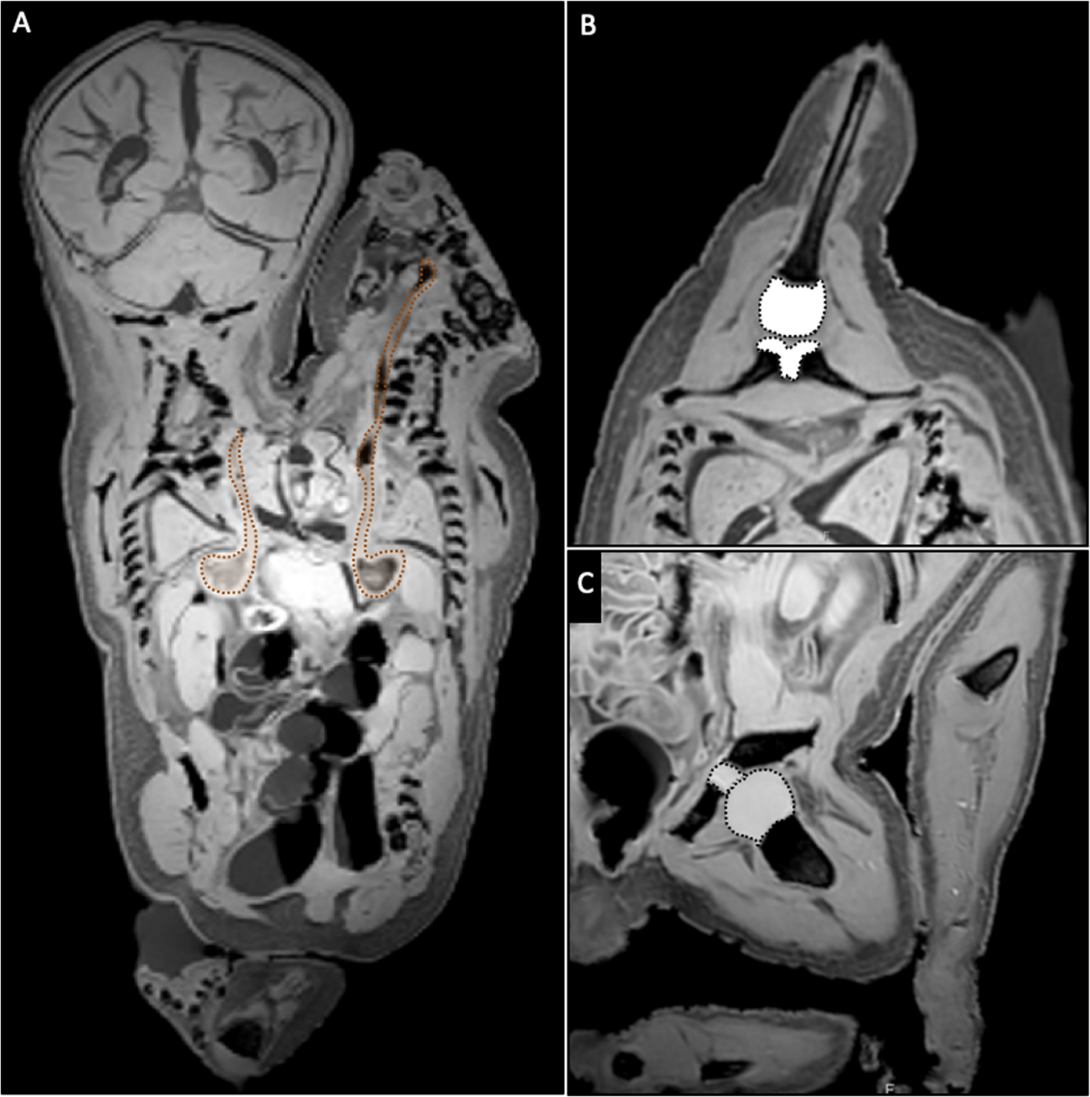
a Coronal T1-weighted magnetic resonance (MR) image showing two separate esophagi and mirror-imaged stomachs underneath an overarching diaphragm (*brown dotted line*). b Axial T1-weighted MR image showing the hypoplastic bone in the third upper extremity articulating with a cartilage tissue mass positioned between the left and right scapulae of the right and left twins, most likely representing a glenoid. c Oblique coronal T1-weighted MR image showing the femoral bone in the third lower extremity articulating with a cartilage tissue mass positioned between the left and right iliac bones of the right and left twins, most likely representing an acetabulum
